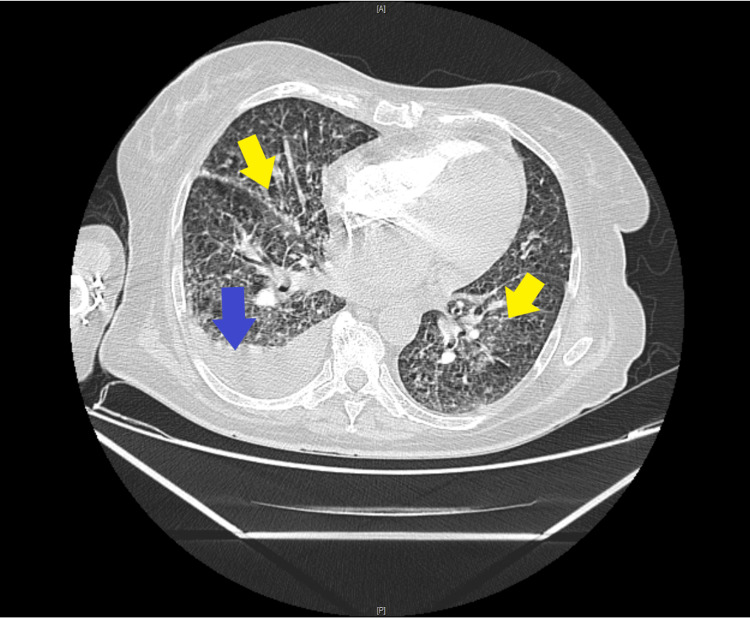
Axial computed tomography images of thorax showing bilateral nodules and micronodules with a miliary appearance (yellow arrow) and a moderate pleural effusion on the right side (blue arrow), suggestive of tuberculosis
